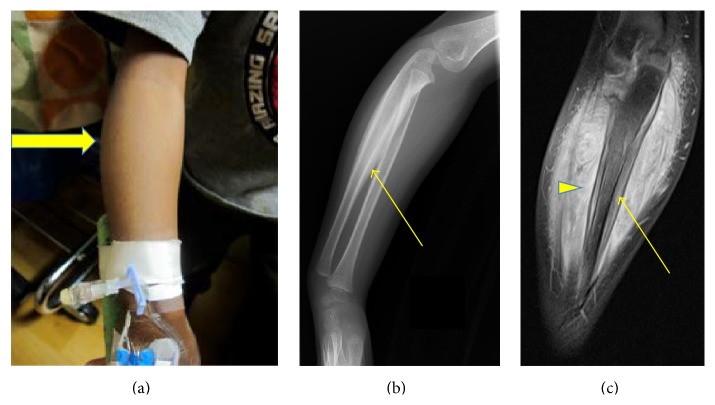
Physical findings and imaging of right arm at diagnosis. (a) Right forearm hypertrophy (yellow arrow). (b) Plain (lateral) radiograph of the right upper extremity revealing diffuse periosteal thickening of both the bones of the forearm (thin yellow arrow) without any focal lytic or sclerotic lesions. The extent and the degree of the involvement of the right ulna were marked as compared to the radius. (c) MRI T2 weighted images of the right forearm demonstrated marked diffuse, circumferential periosteal thickening involving the radius and ulna. Ulnar involvement (thin yellow arrow) was severe as compared with the radius. No focal osseous lesion was identified. Also notable was marked soft tissue involvement including the muscular compartment consistent with myositis (arrowhead).
